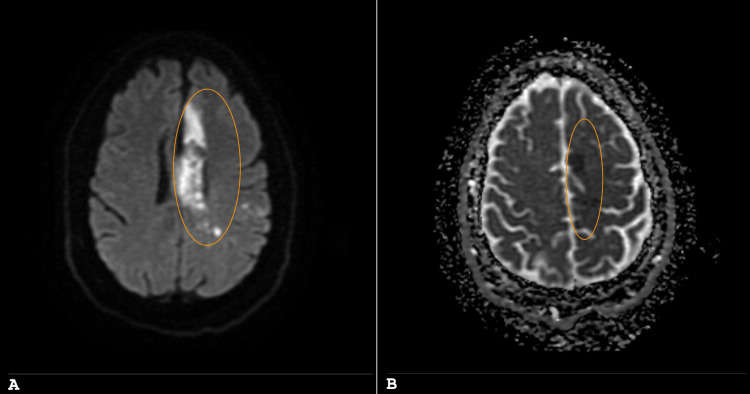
MRI of the brain. Panels A (diffusion-weighting imaging) and B (apparent diffusion coefficient mapping) demonstrate multifocal areas of restricted diffusion noted along the median left frontal cortex in the ACA distribution, as well as throughout the posterior left frontal and left parietal cortices (circled in orange). ACA: anterior cerebral artery.
